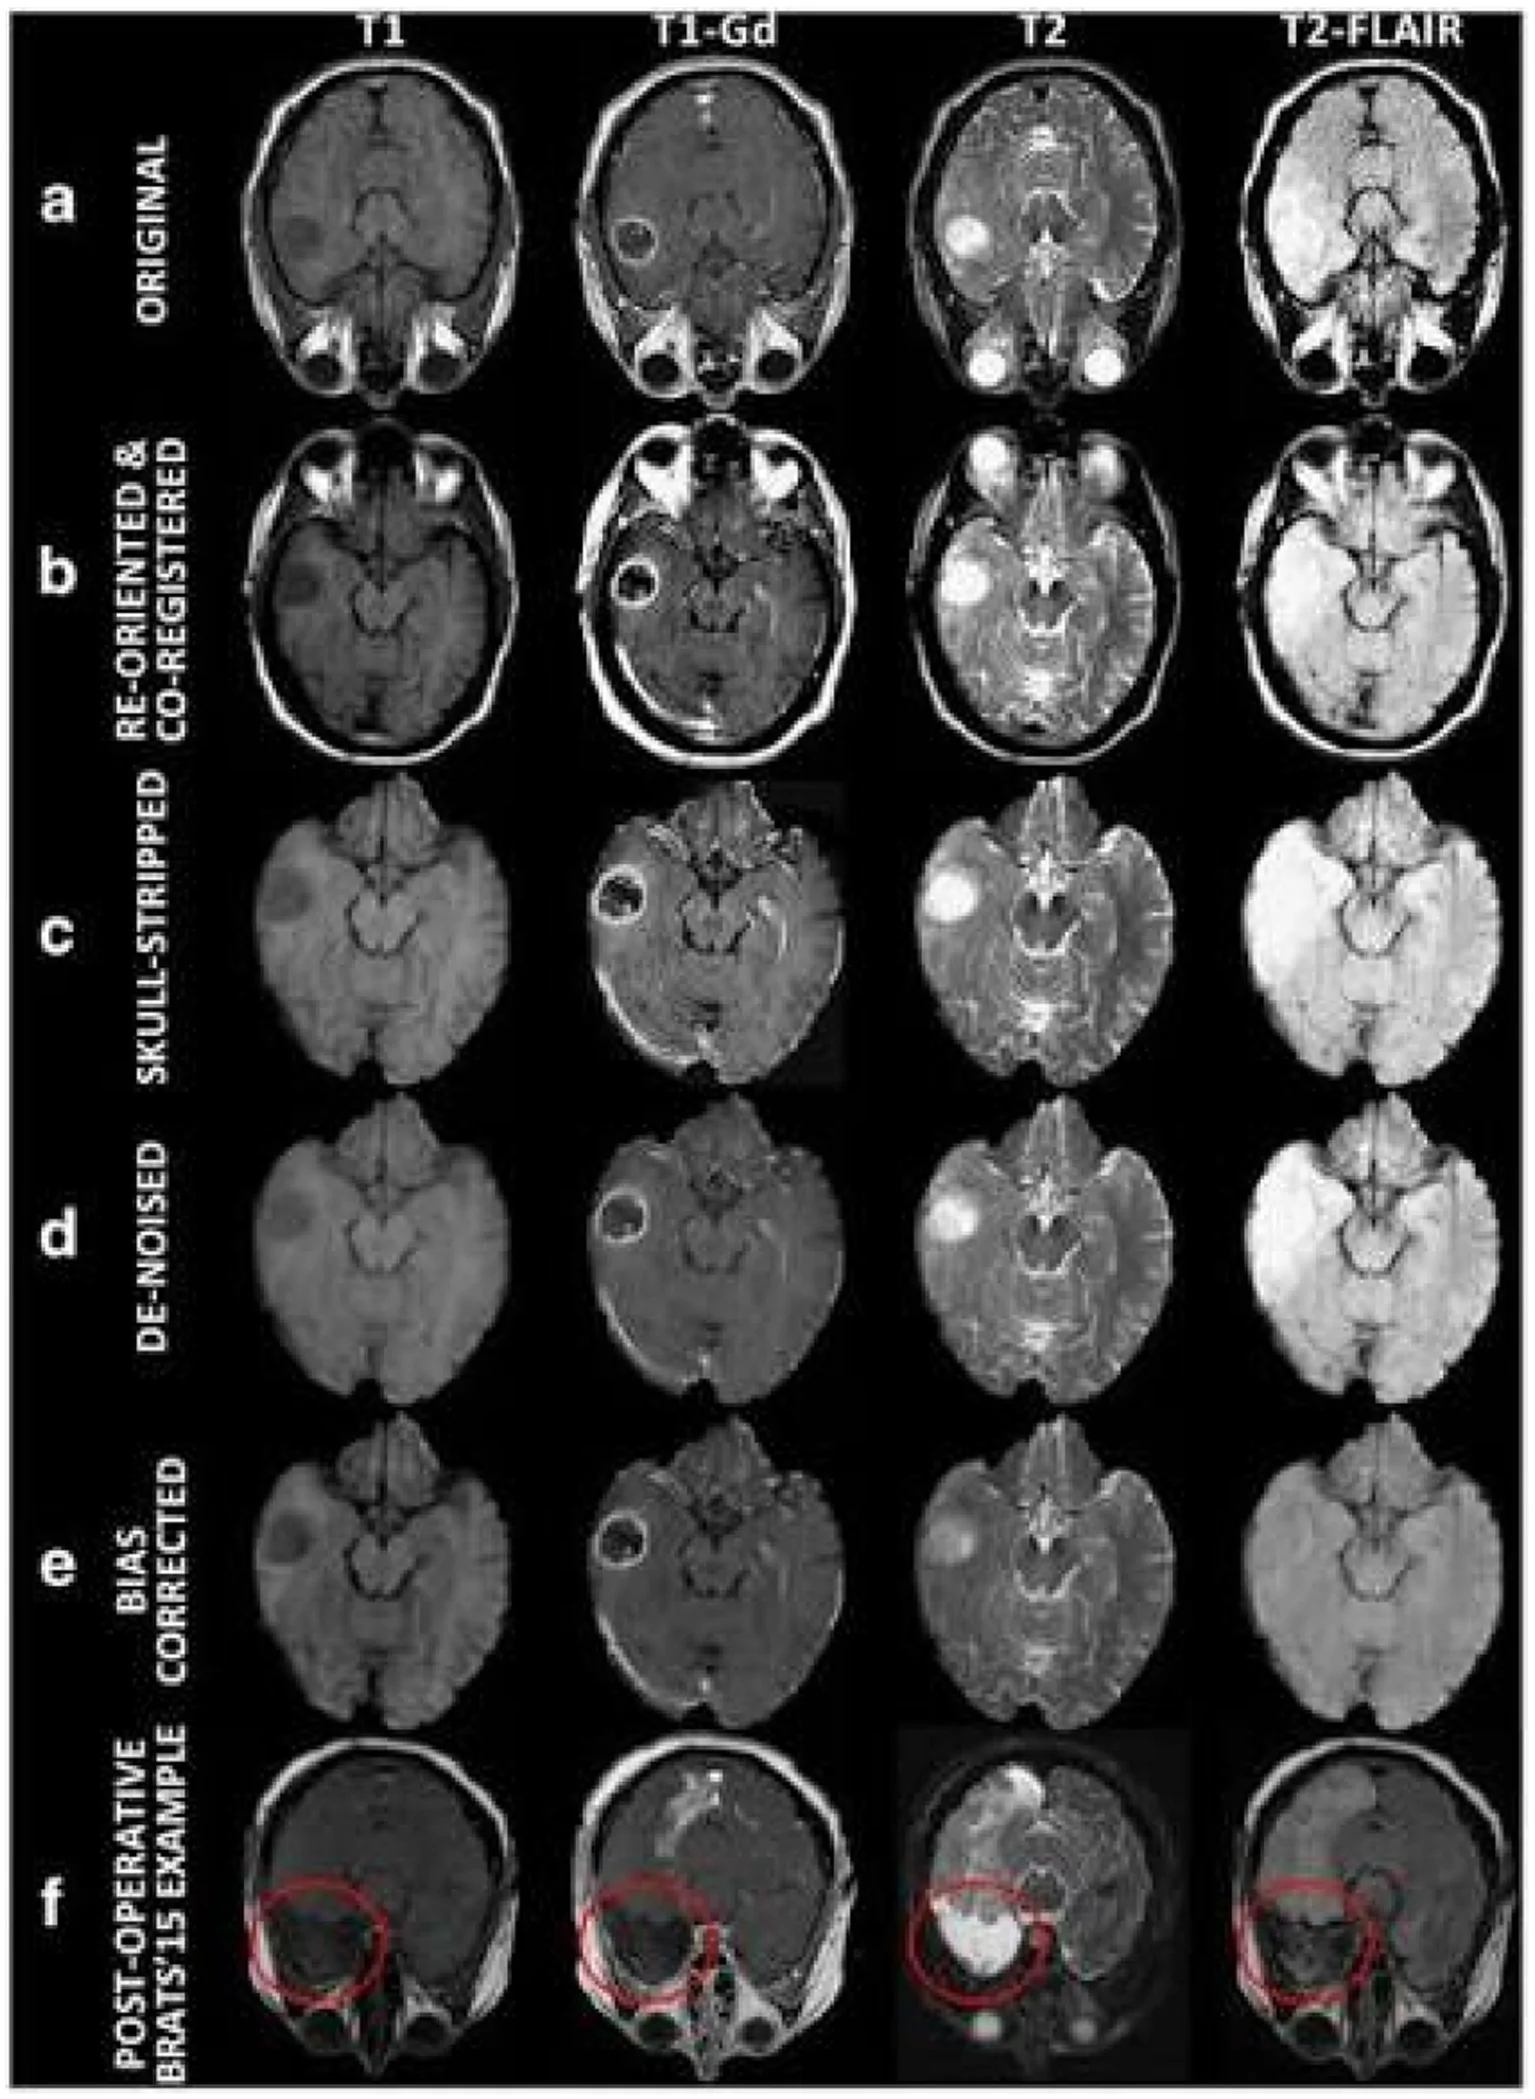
Examples of the original, pre-processed, and post-operative MRI volumes are presented, with intensity non-uniformity correction omitted because it degraded the T2-FLAIR signal (84). (a) original MRI volumes; (b) re-oriented and co-registered; (c) skull-stripped; (d) de-noised; (e) bias-corrected images across T1, T1-Gd, T2, and T2-FLAIR imaging types; and (f) post-operative examples with red circles highlighting areas of interest.
